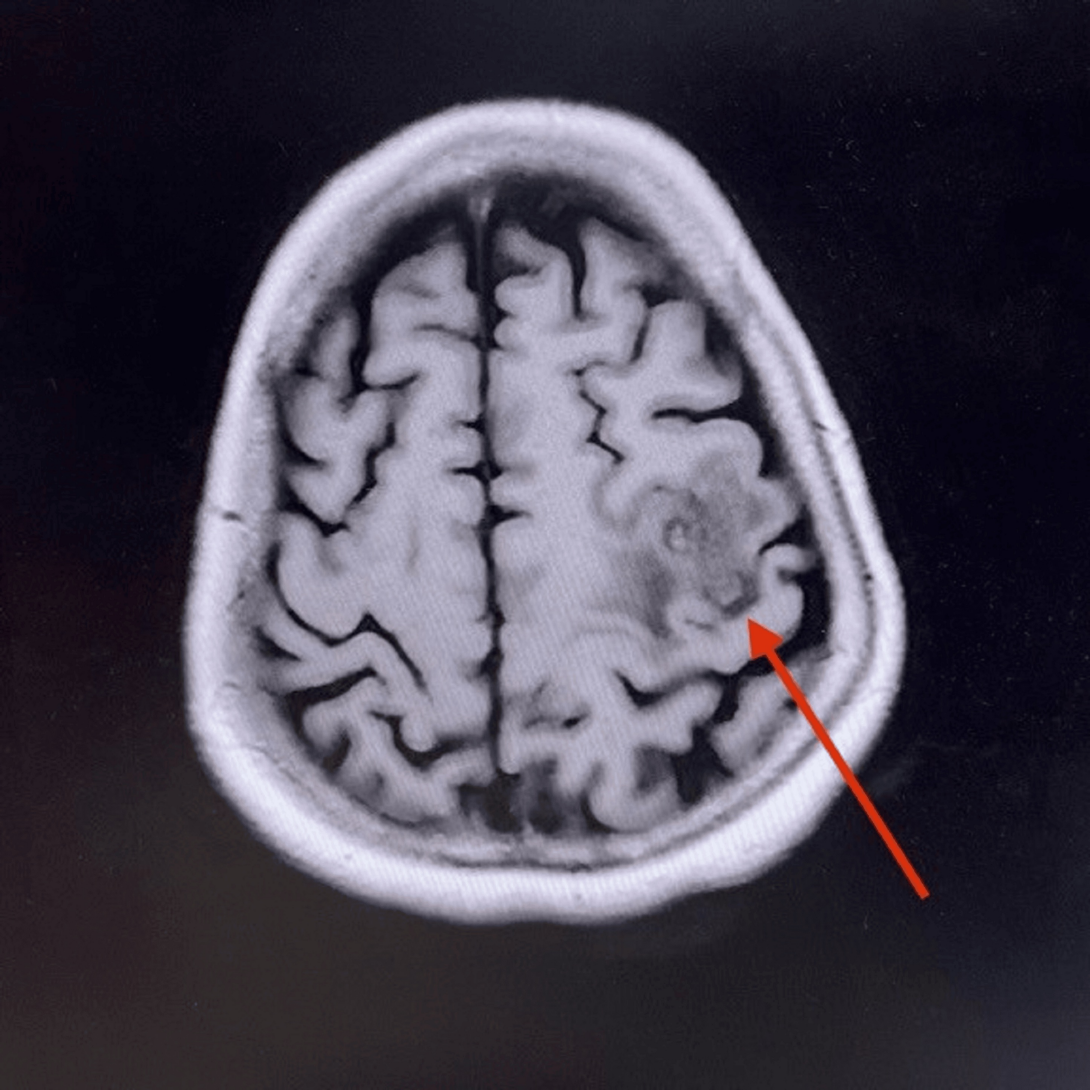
Sagittal section of the patient’s brain contrast MRI showing brain abscess in left frontal lobe (24 mm × 15 mm × 17 mm) (red arrow) and a further small area of early abscess formation in the right posterior temporal lobe.
